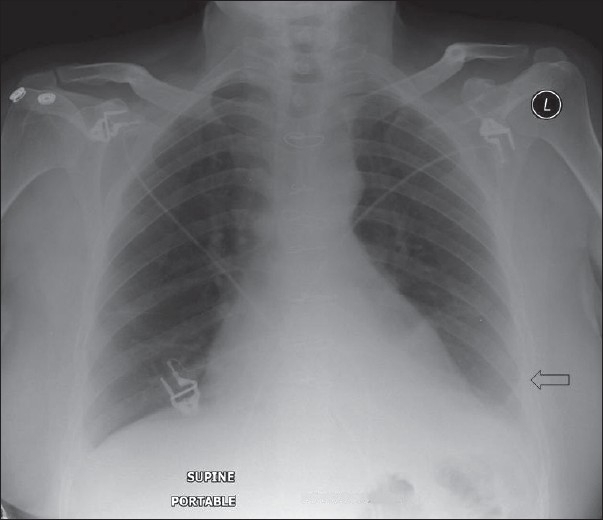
An AP chest radiograph on a 55-year-old female immediately following CABG, shows hazy opacification at the lung base and blunting of the left costophrenic angle due to a small pleural effusion, which is a common feature following thoracotomy (black arrow)
